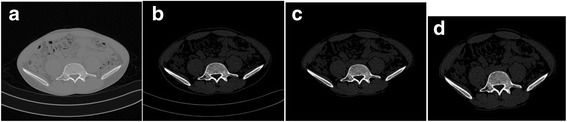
The pre-processing results of Patient 1. (a) is the original CT image whose pixel plane is 512 × 512. (b) is the image obtained by windowing, the window width is 600 Hu, and the window level is 900Hu. (c) is the pelvis area without the CT table, cables, etc. (d) is the pre-processed image. The image size is adjusted by cutting out the non-pelvis area as much as possible
